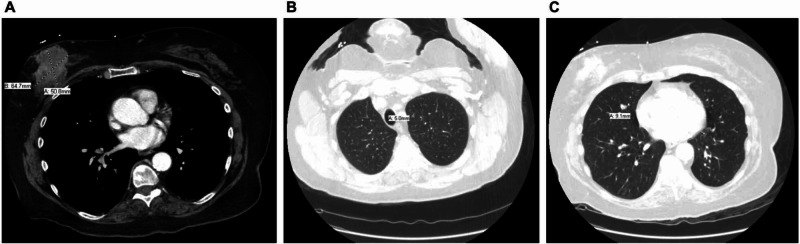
Various CT scan views display (A) right breast mass (axial view) and (B and C) pulmonary nodules (axial views).
